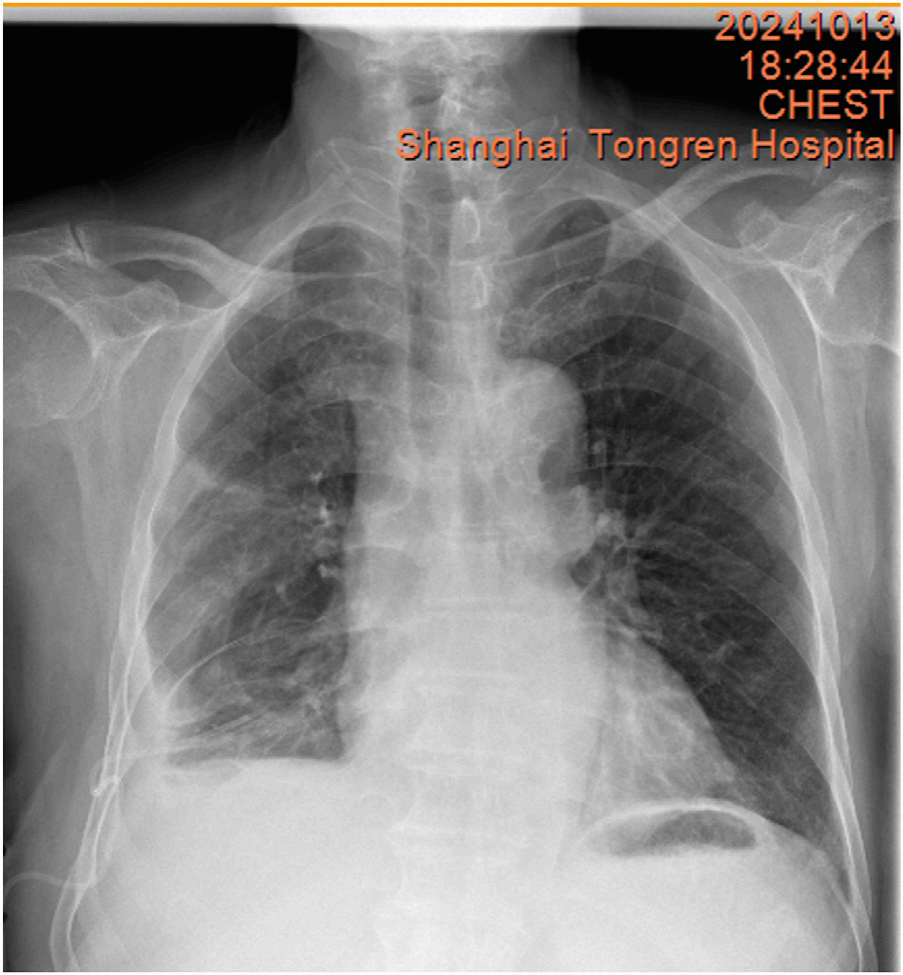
Chest X-ray on 13 October 2024, showing a decrease in right pleural effusion.
